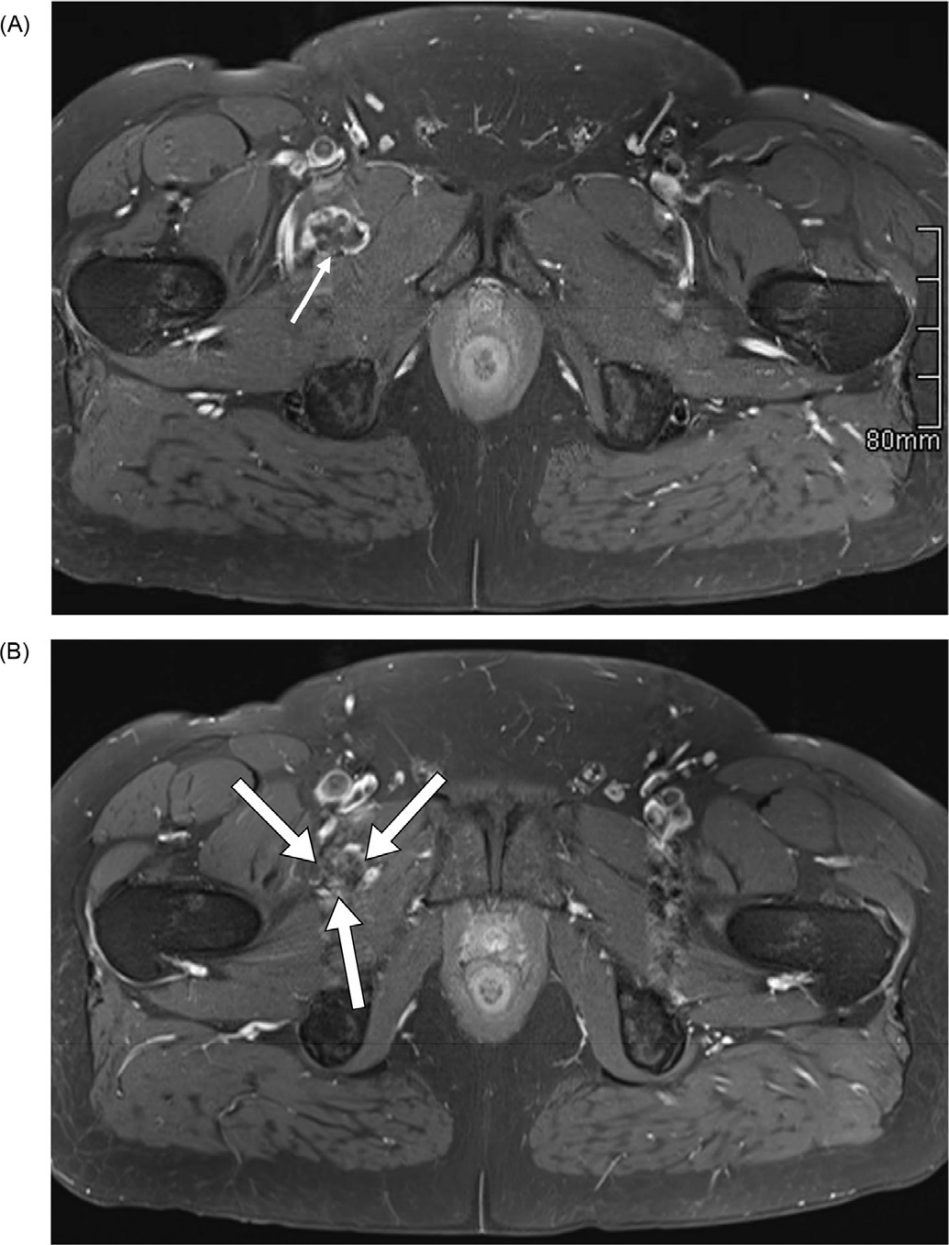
(A) and (B) Selected postcontrast fat-saturated T1 MRI pelvis axial slices of the same patient demonstrate a peripheral and septal enhancement pattern of the right groin lesion (arrows) with no central enhancement.
